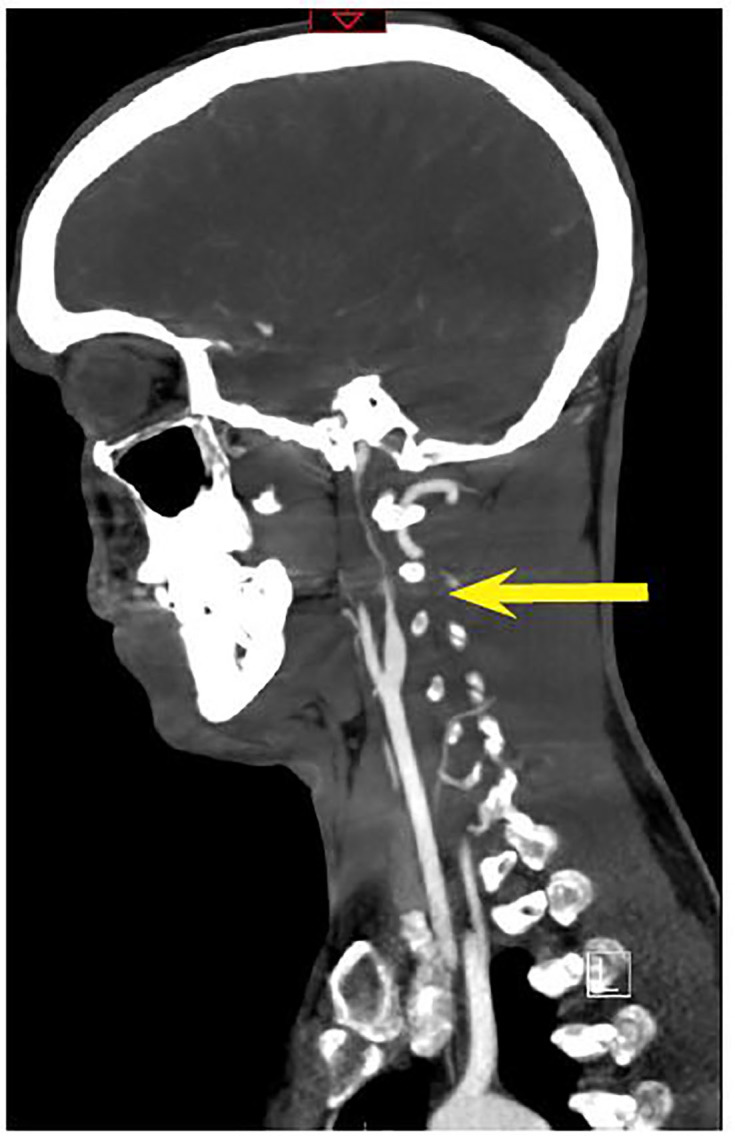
CTA sagittal view showing “carotid string sign” (yellow arrow) referring to the thin string of intravenous contrast material distal to the stenotic focus in the internal carotid artery.
